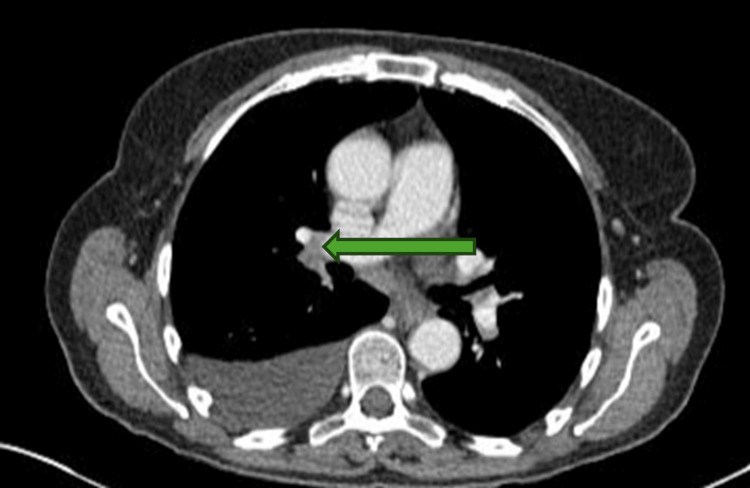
CT thorax axial section showing a hilar lymph node measuring 1.3 cm (green arrow)
